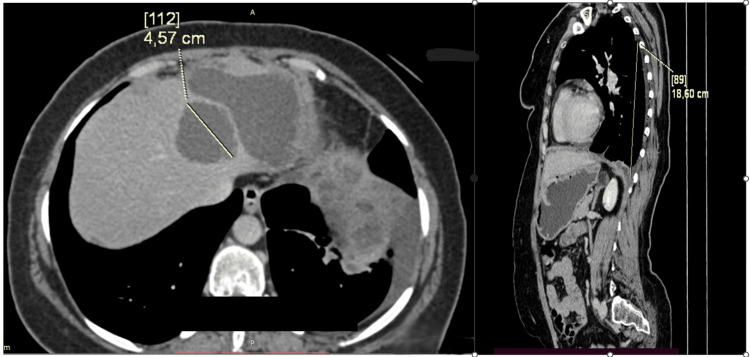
Representative imaging during the treatment course. Follow-up CT (Day 540) demonstrating radiographic progression of metastatic disease after sequential therapy. After initial response followed by approximately seven months of stable disease on pazopanib and subsequent re-induction, progressive disease was documented on Day 540.
